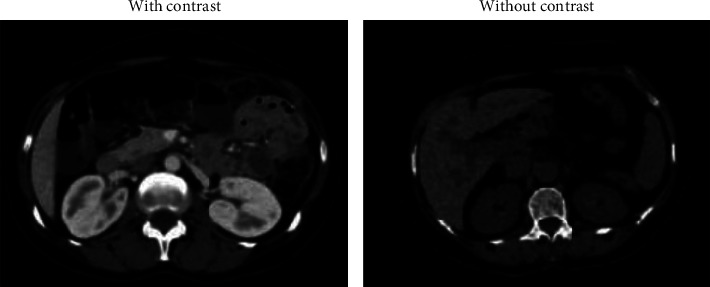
Sample CT scans taken from the dataset.
